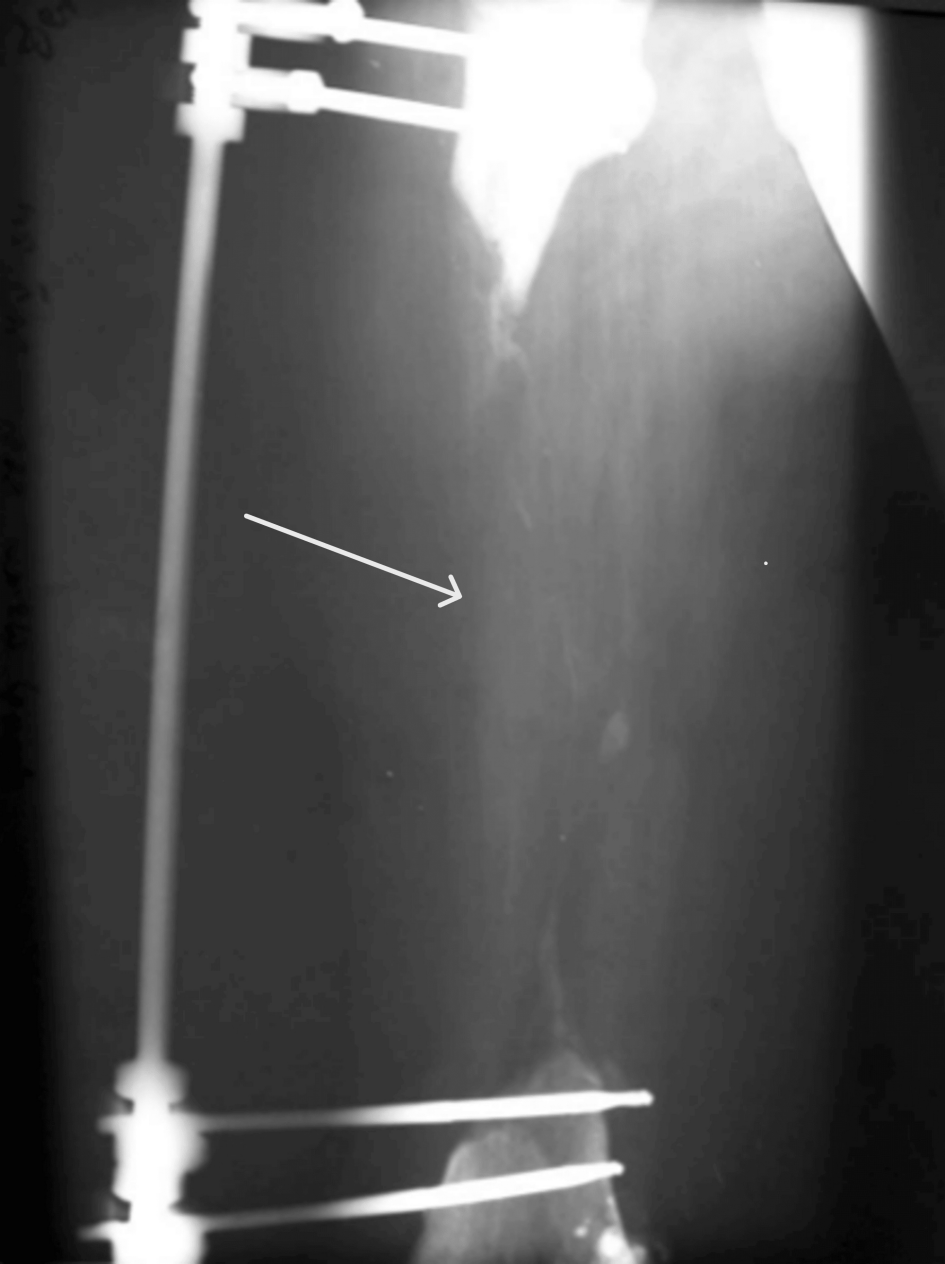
X-ray image depicting femoral diaphysis defect following the removal of diaphyseal fragments. Missing femoral diaphysis is indicated by the arrow.
